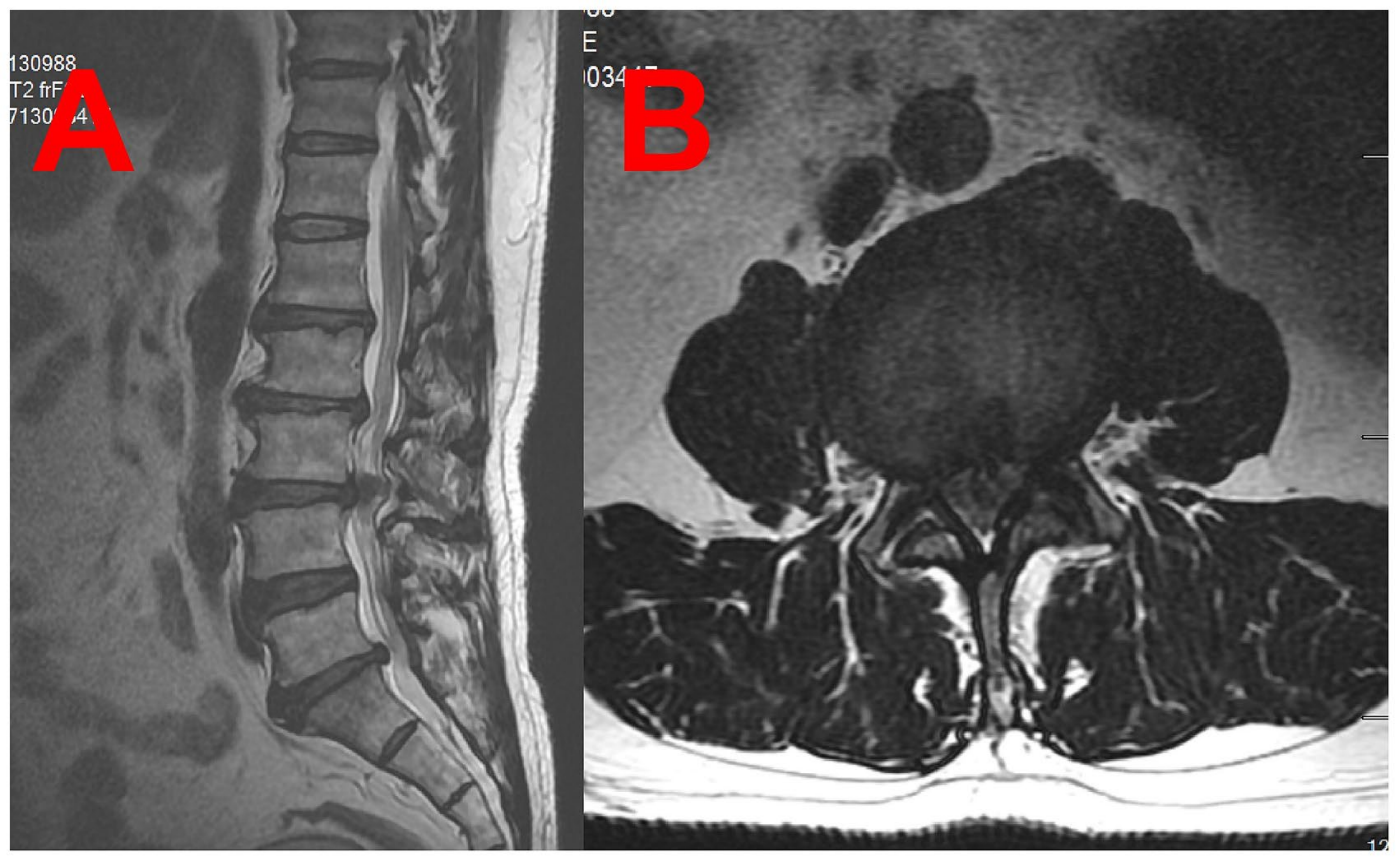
(A,B) Lumbar MRI revealed a herniated disc between L3 and L4, compressing the dural sac.
